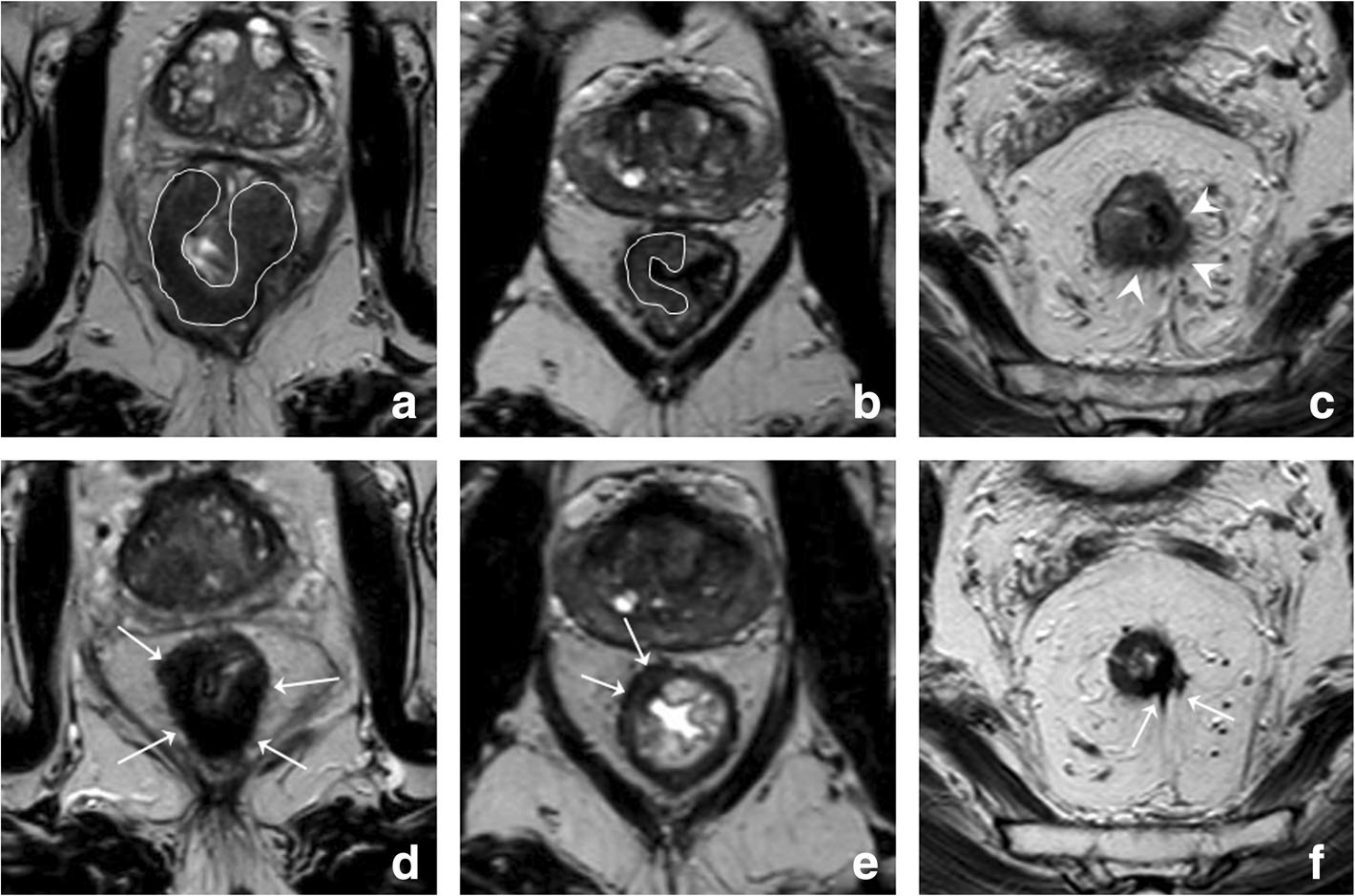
Patterns of fibrosis. Examples of the pre-CRT (a–c) and corresponding post-CRT (d–f) T2-weighted images of three male patients with low to mid-rectal tumors. The first patient has a well-defined, almost circular tumor mass (a). After CRT (d), the tumor has undergone fibrotic transformation that follows the shape of the primary tumor, resulting in a full thickness (semi) circular fibrotic wall thickening. The second patient has a relatively small, semicircular tumor (b). After CRT, only a small focal area of fibrosis remains visible that is confined to the rectal wall (e). The third patient has an irregularly shaped, spiculated tumor (c). After CRT, the fibrosis also has an irregular aspect with persistent spiculations (f)
